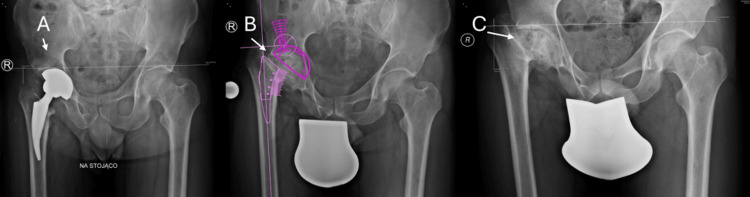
Standing Pelvic Radiographs Demonstrating Post-THA Anatomy and Preoperative Biomechanical Context A: Standing anteroposterior pelvic radiograph demonstrating postoperative alignment after right total hip arthroplasty (THA).
